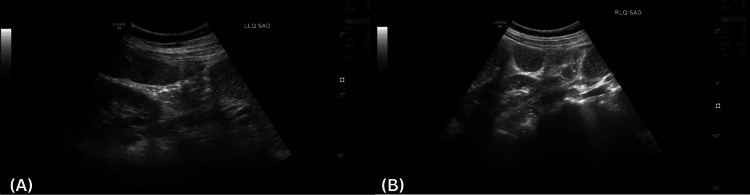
Sagittal sonographic view of the (A) right and (B) left lower abdominal quadrants demonstrating distended bowel loops measuring 3-3.5 cm in diameter.
